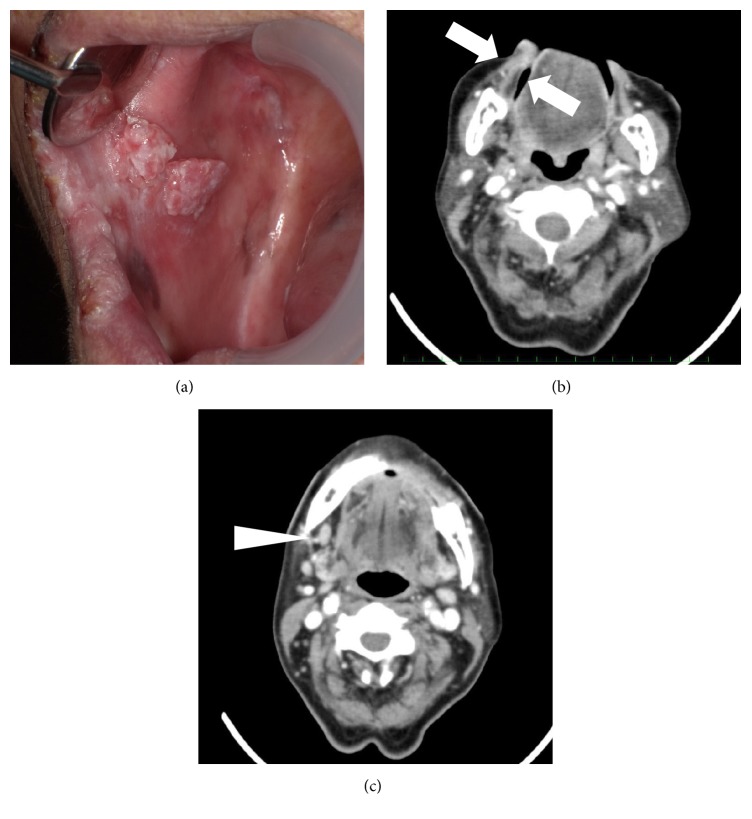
Intraoral findings at most recent follow-up*. Intraoral findings (a) and CT images of the right buccal region (b, arrow) and submandibular lymph node (c, arrow head).
